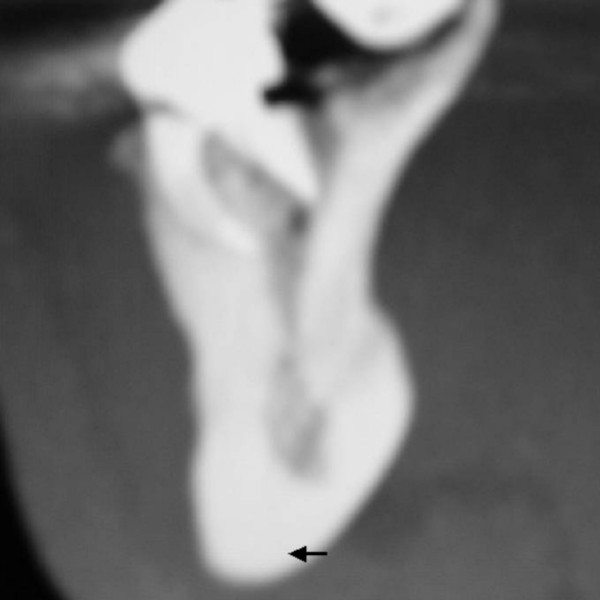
(patient 2). Sagittal reformatted multiplanar computed tomography showed irregular increased density in the mandibular bone and the increased density of cancellous bone at the inferior borders.
